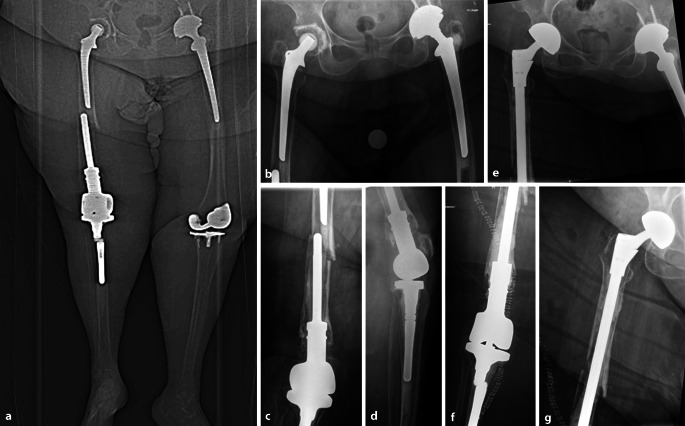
Fallbeispiel: 74 Jahre, weiblich, Body-Mass-Index 47 kg/m^2^. Die Patientin ist im Rollstuhl mobil („low demand“). Der Transfer erfolgte bislang über die linke Seite. Sturzgeschehen beim Aussteigen aus einem Pkw. a Topogramm der CT mit Darstellung des eindrücklichen Weichteilmantels. Es zeigt sich eine interprothetische Femurfraktur (Unified-Classification-System-Typ D) bei einliegender, nicht gelockerter, vollzementierter Hüftprothese und zementiertem distalem Femurersatz. Der interprothetische Abstand beträgt lediglich wenige Zentimeter. Weite Konfiguration des Markraumes mit ausgedünnter Femurkortikalis. b–d Interprothetische Fraktur mit gelockertem distalen Femurersatz bei festsitzender, zementierter tibialer Komponente. Vorbestehende Patella alta. e–g Postoperative Röntgenkontrolle nach Revisionsoperation mit Wechsel auf eine zementfreie tripolare Hüftgelenkpfanne (Fa. Link, Typ BiMobile) sowie Implantation einer Durchsteckprothese mit Erhalt der tibialen Komponente (Fa. Implantcast, Typ MUTARS (Fa. Implantcast, Buxtehude, Deutschland)). Postoperativ wurde die schmerzadaptierte Belastung freigegeben
